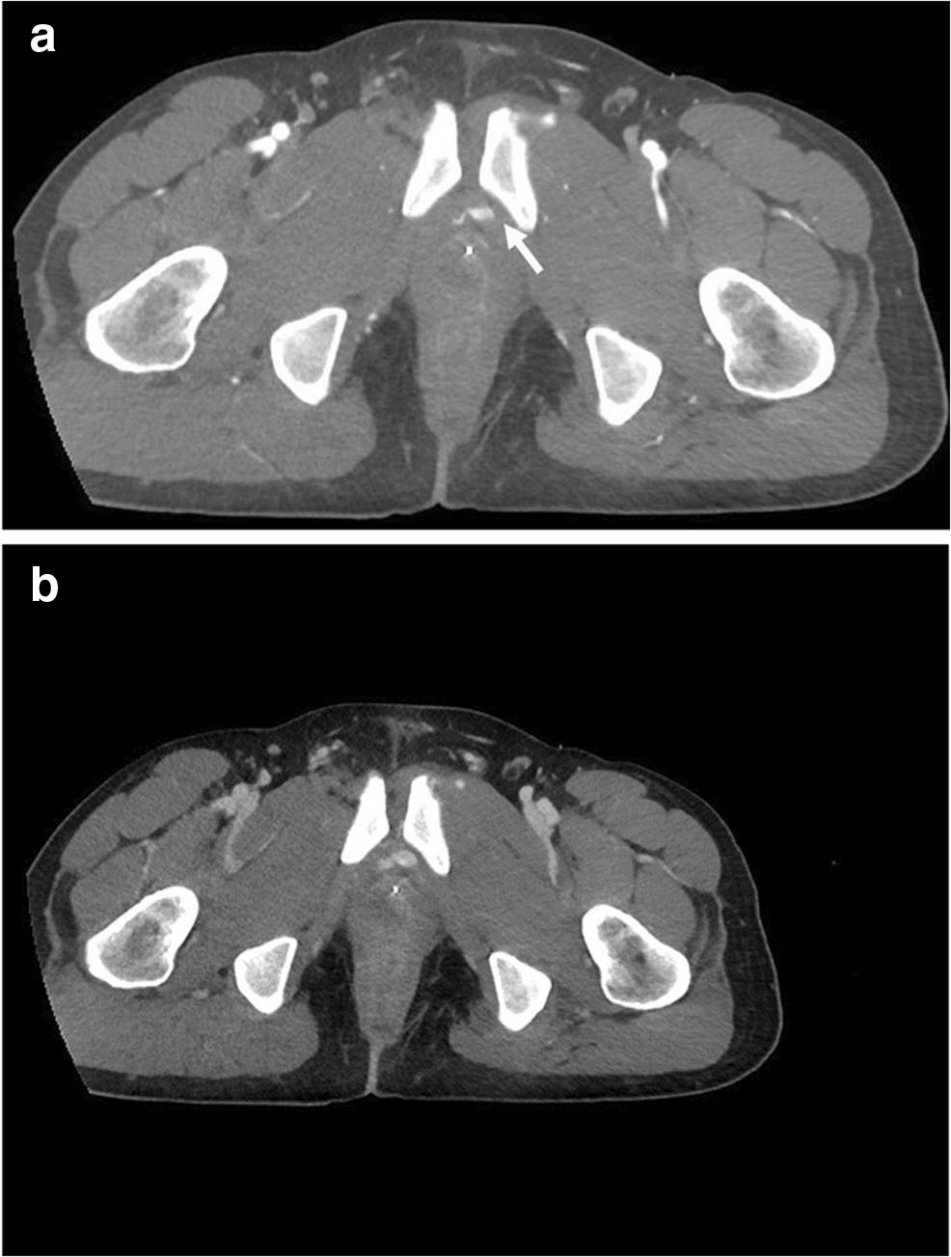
Extravascular area of hyperattenuation from the left pudendal vascular territory (white arrow) in arterial phase (a) and portal venous phase (b) CT
